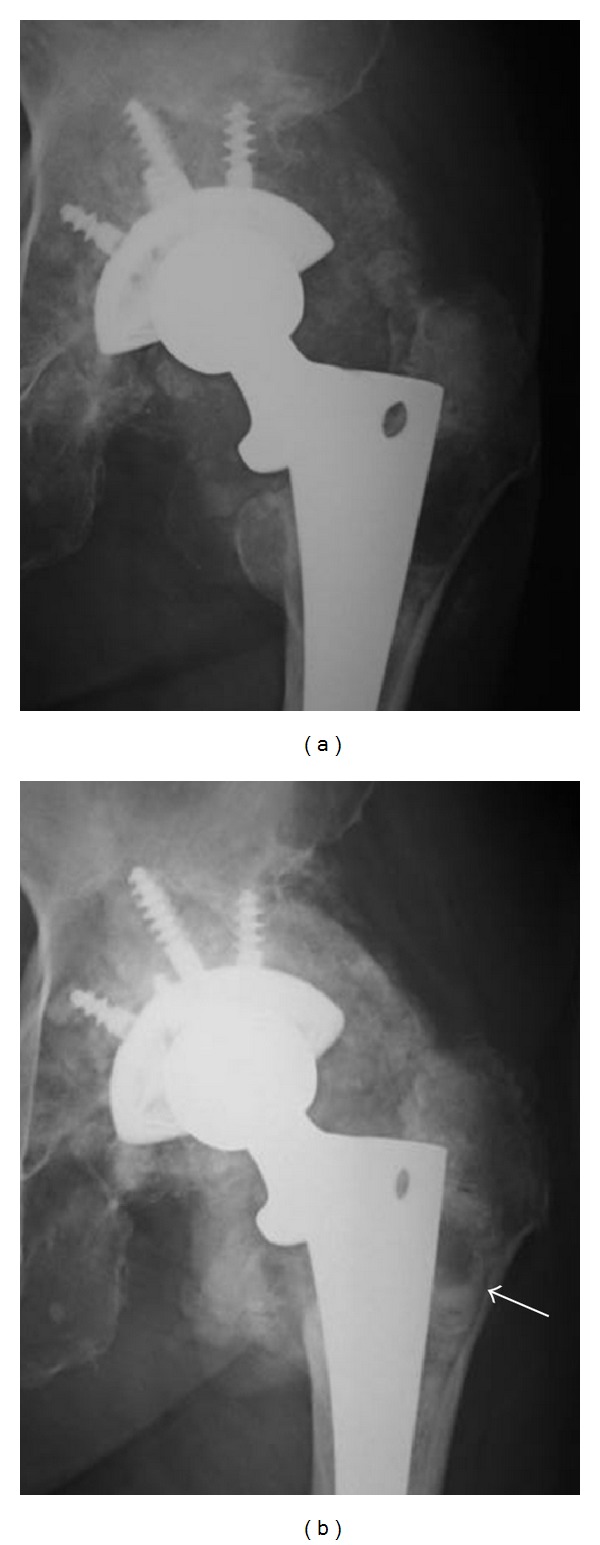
Comparison of X-ray findings at the initial diagnosis (a) and 1 year later (b). Calcification around the left hip prosthesis was observed. The arrowhead indicates niveau formation in the greater trochanter marrow cavity.
